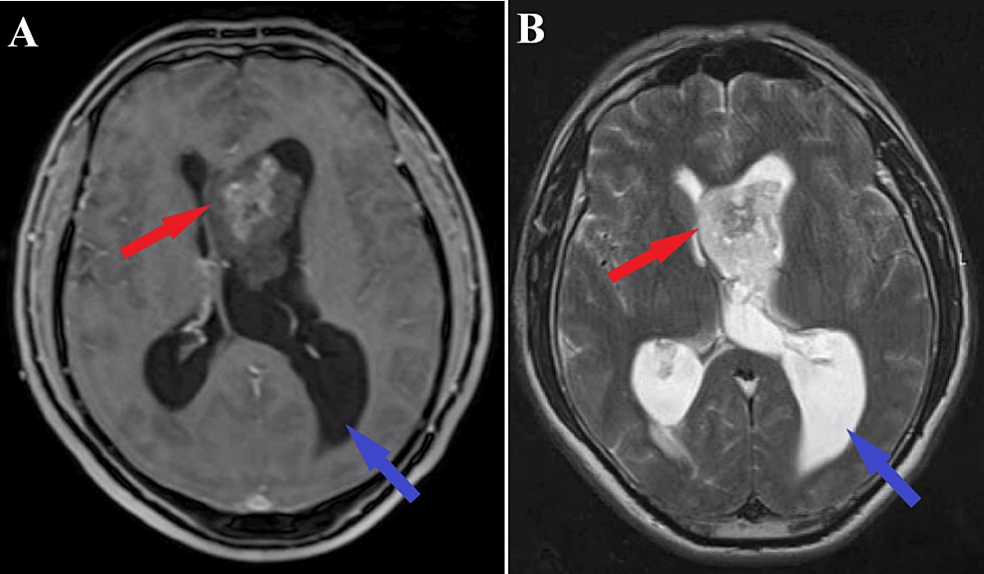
Preoperative T1Gd and T2 sequence MRI The axial section of MRI T1 sequence with gadolinium (A) and axial section of MRI T2 sequence (B) depict the intraventricular tumoral process (red arrows) with the associated significant obstructive hydrocephalus (blue arrows)
